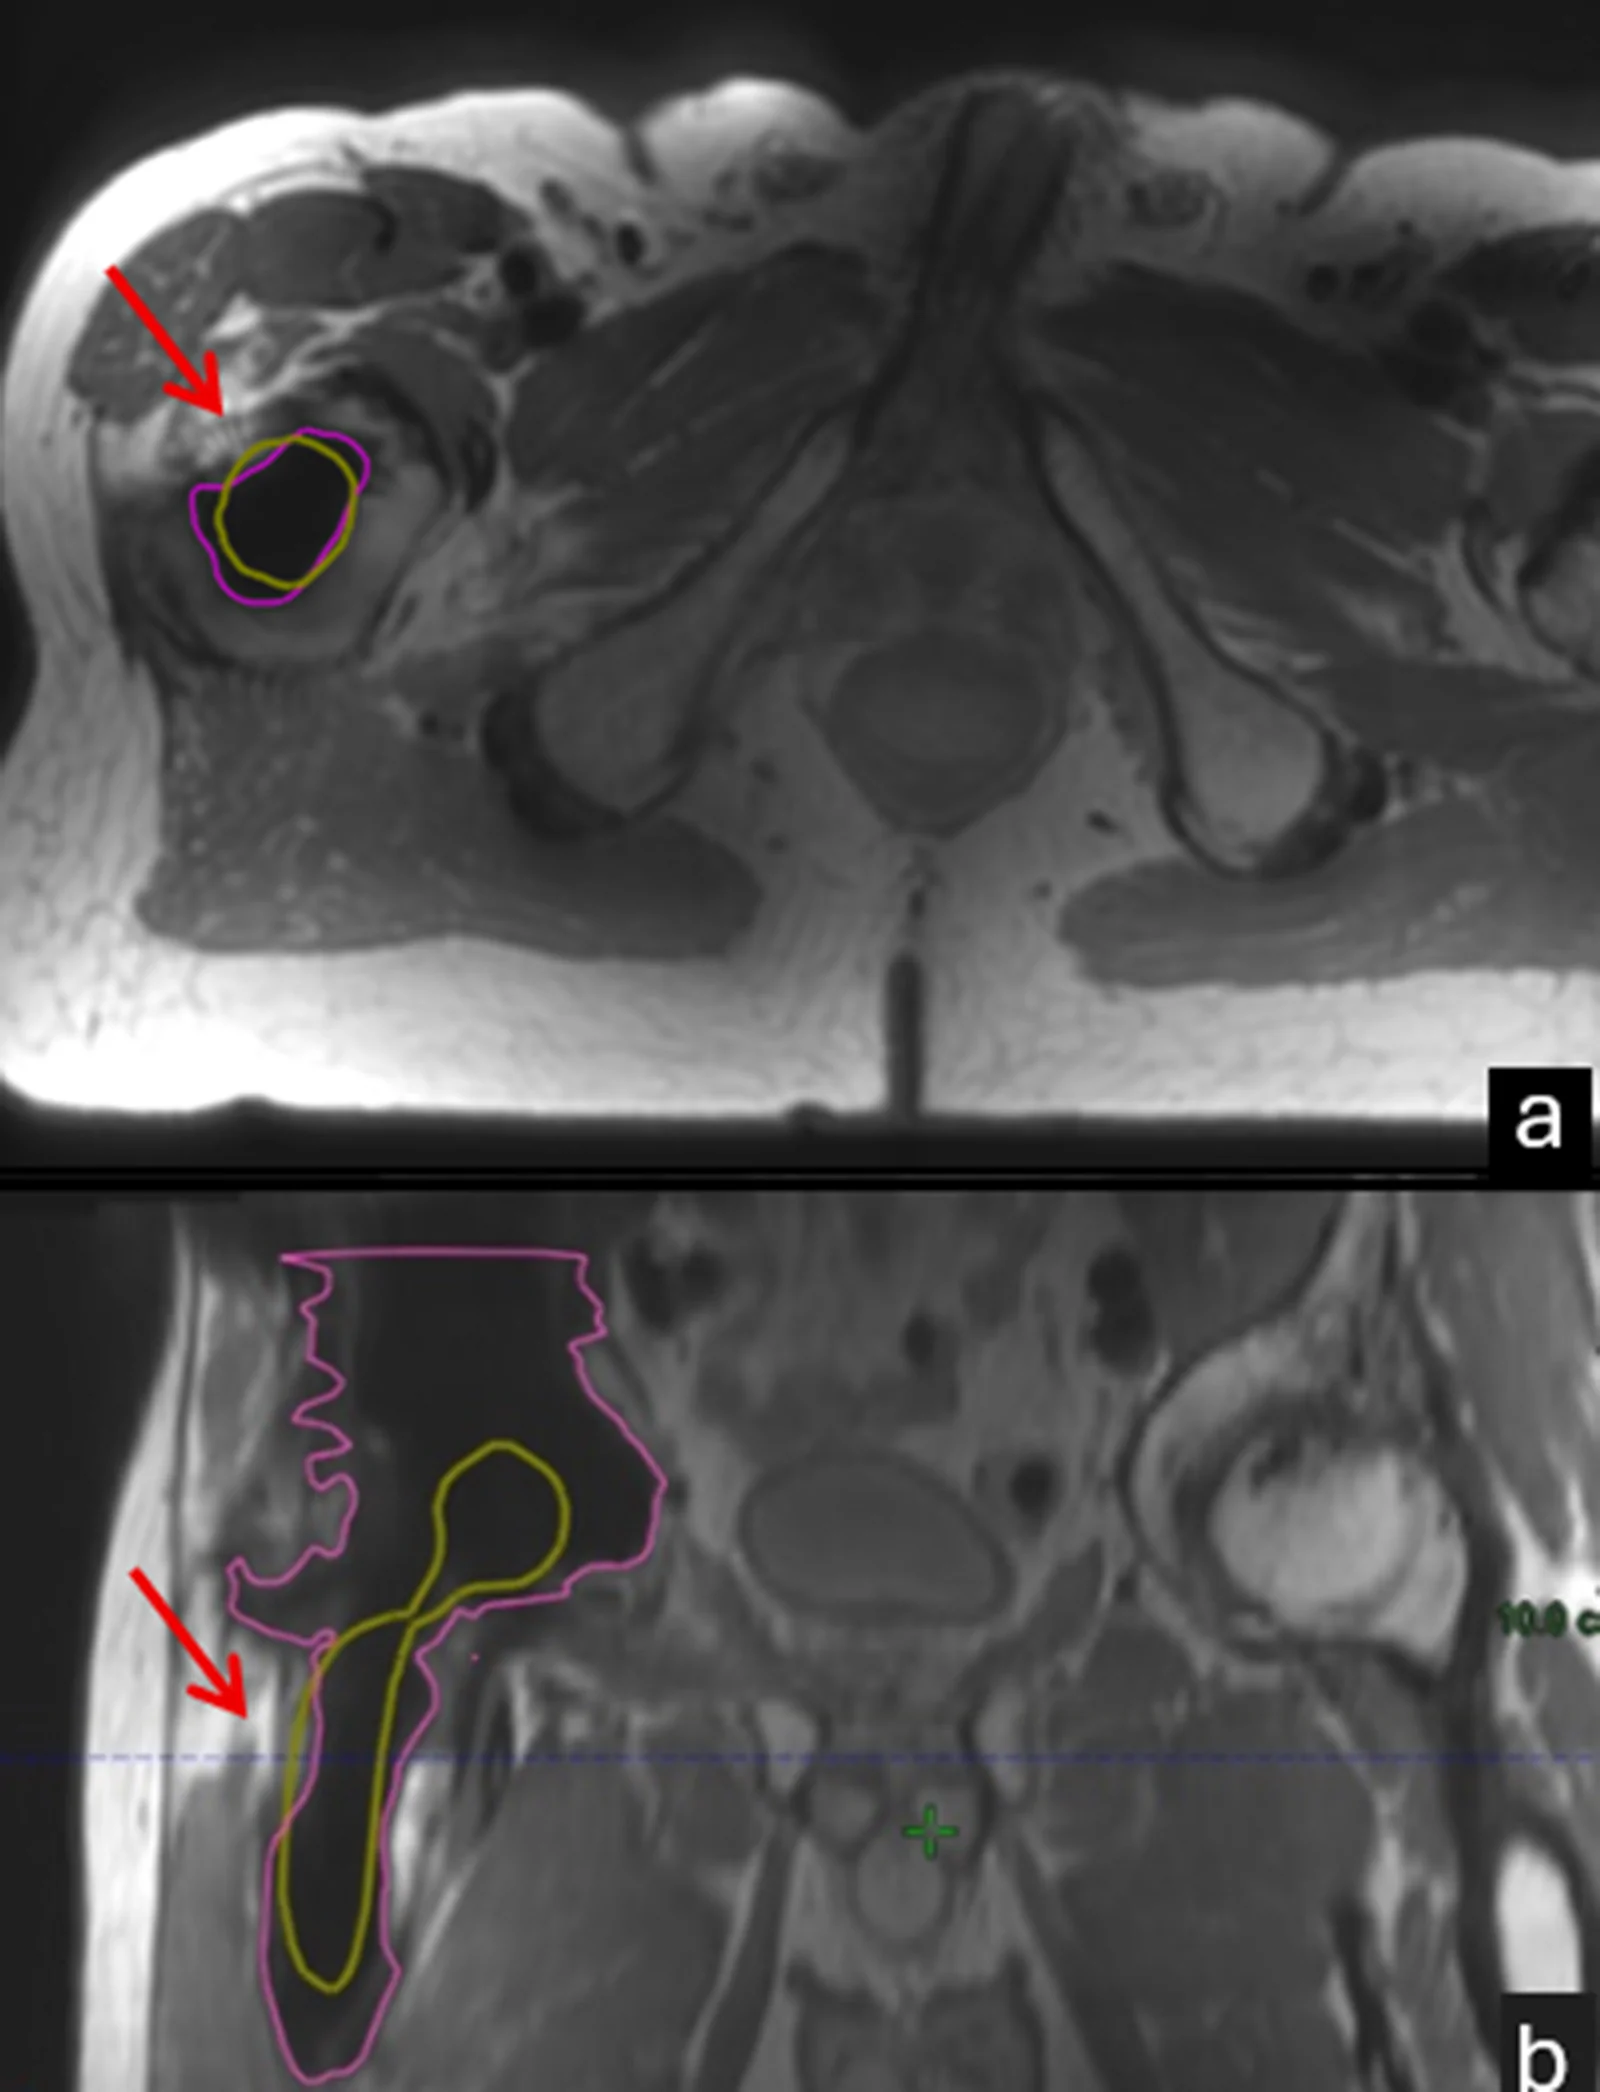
MAVRIC-SL image with the signal void delineated (pink contour). The contour of the hip prosthesis (yellow contour) was delineated on the CT image and transferred to the MR image after a focused prosthesis-region registration. (a) Axial plane. (b) Coronal plane. Note that the hip prosthesis extends beyond the signal void void along its distal stem, inferior to the femoral head, as indicated by the red arrows
